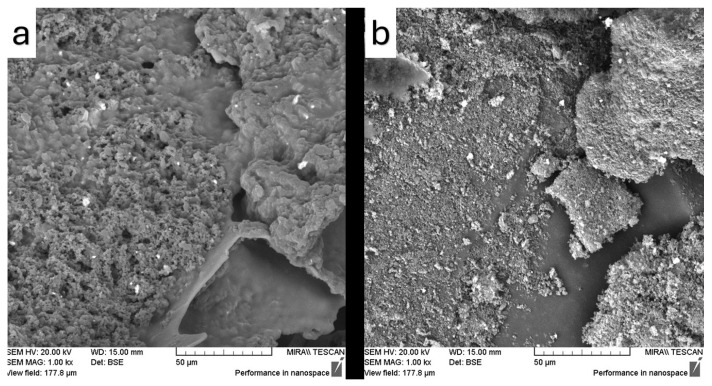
SEM images of (a) ultrasonicated fenbendazole dried using the sample concentrator (FenSon3SC), 1000× magnification; and (b) ultrasonicated fenbendazole, which was freeze-dried (FenSon3FD), 1000× magnification.
